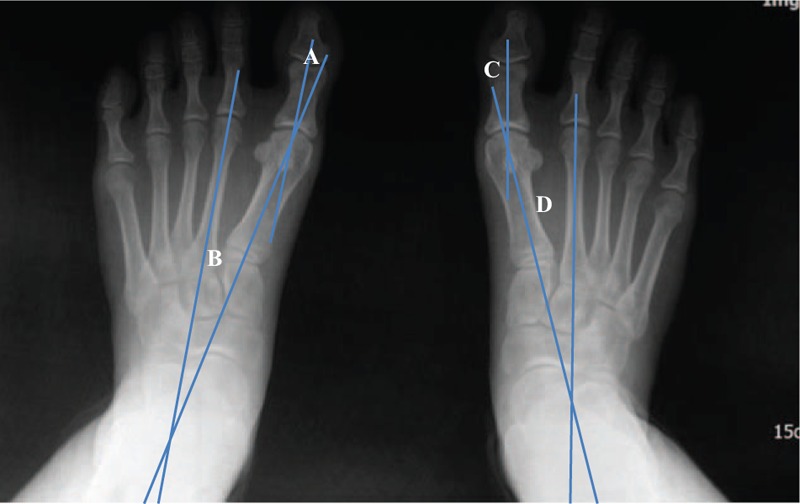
Final weight bearing radiographic view of both feet (A, left hallux valgus angle; B, left intermetatarsal angle; C, right hallux valgus angle; D, right intermetatarsal angle).
